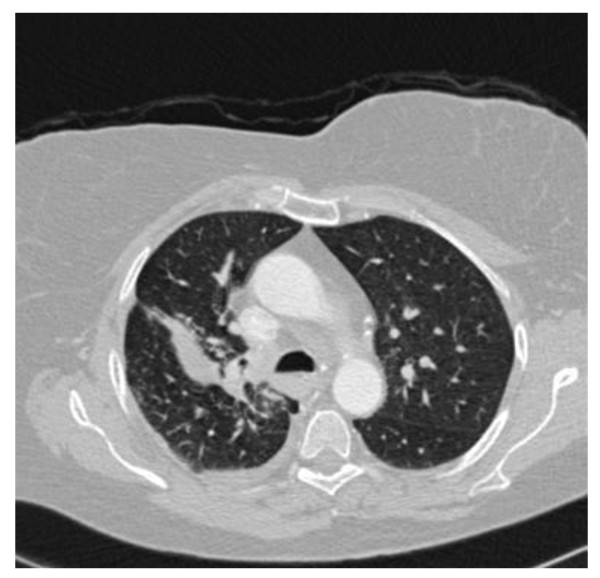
High-resolution computed tomography scan of the chest (upper-lung zones). Radiological improvement was achieved after treatment with steroids was commenced.
